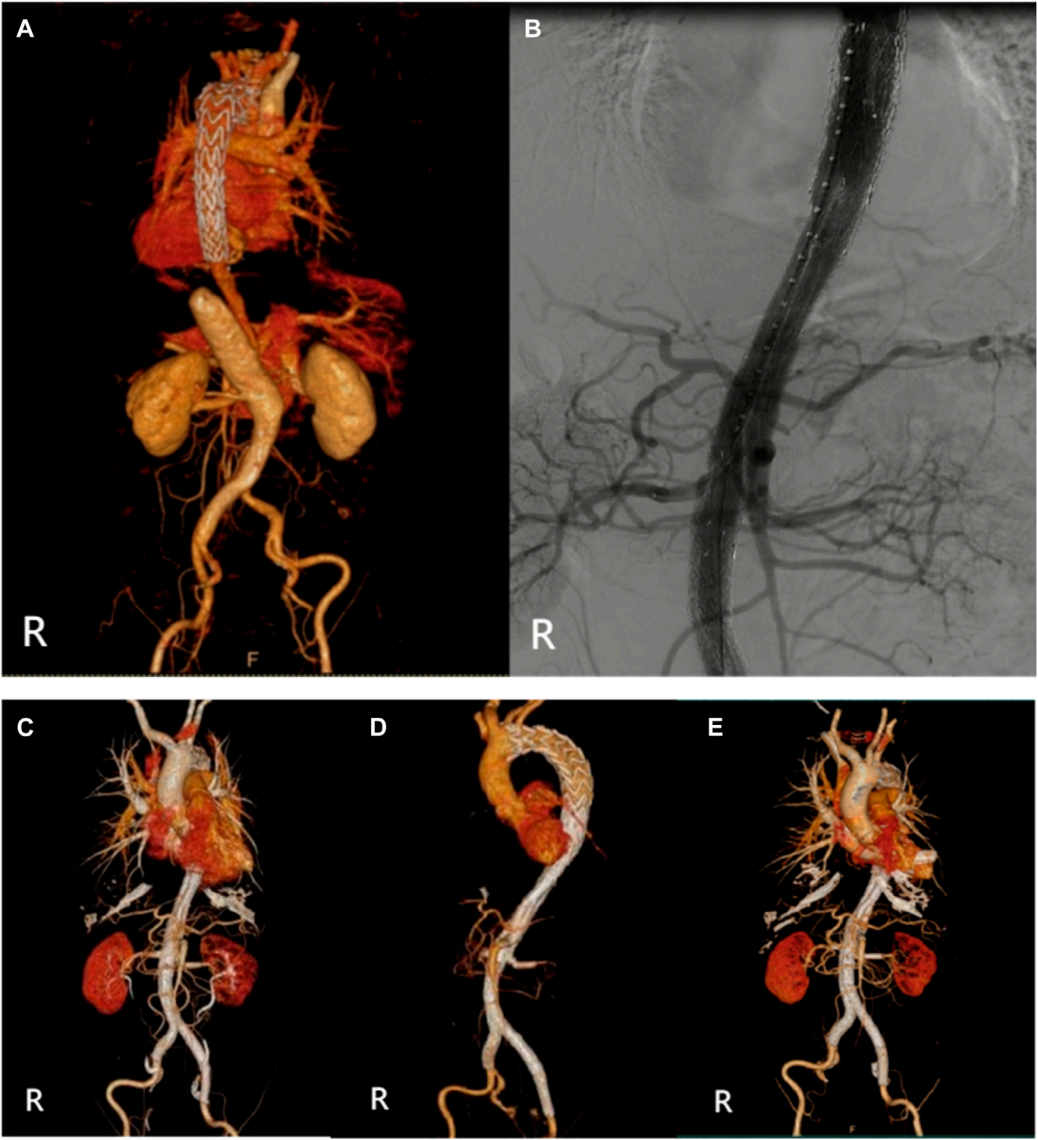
(Case 1) (A) Preoperative CTA. (B) Intraoperative imaging. (C) Postoperative CTA (1st week). (D) Postoperative CTA (6th month). (E) Postoperative CTA (24th month).
